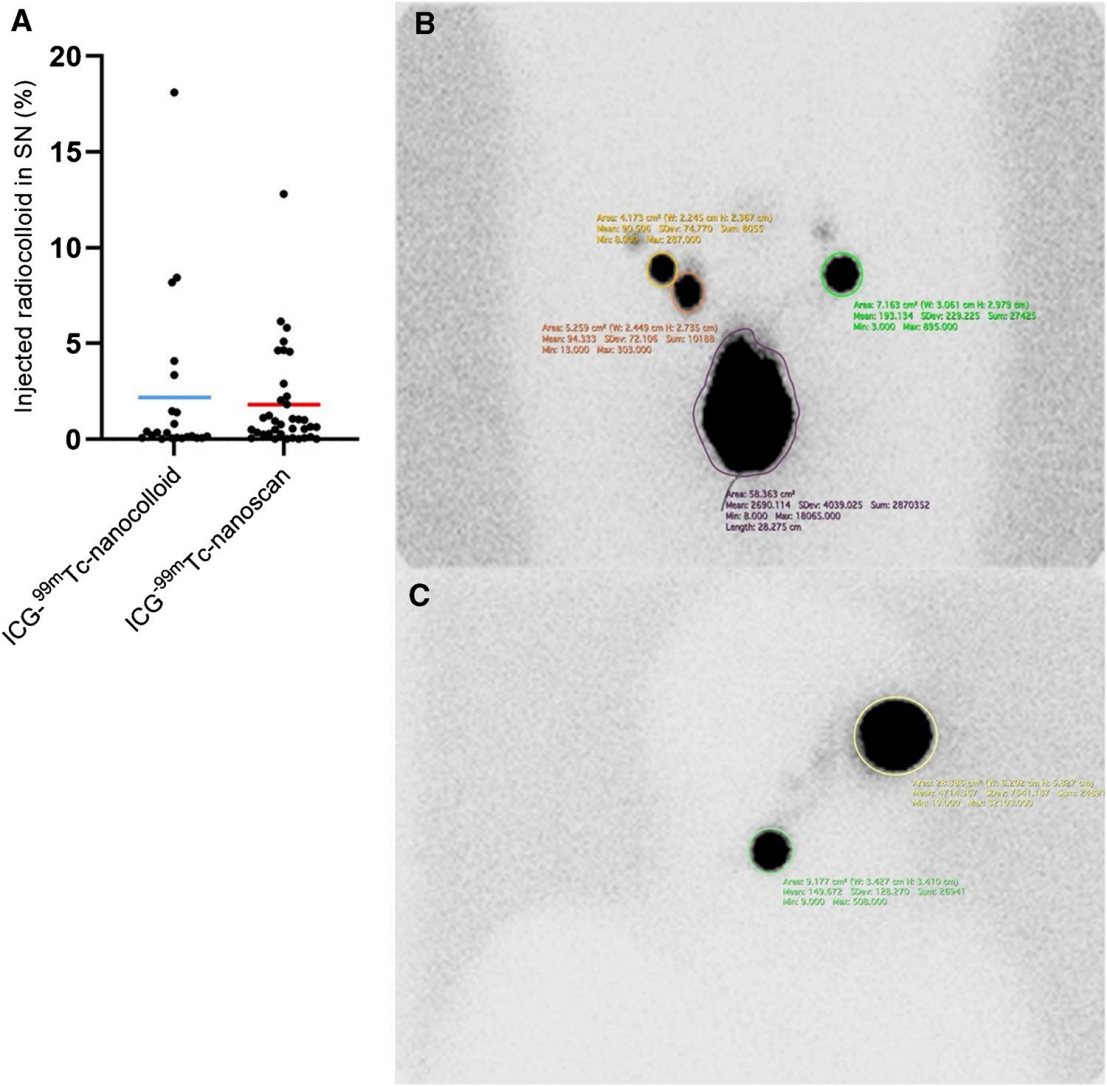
The uptake of injected radiocolloid in SNs on planar lymphoscintigraphy. A There is no difference in uptake when ICG-^99m^Tc-nanocolloid (*blue line*) and ICG-^99m^Tc-nanoscan (*red line*) are compared. B Example of analyses in Horos in a patient with PeCa; ROIs around the injection site (*purple circle*) and SNs (*green, orange and yellow circles*). C And the analysis of a patient with melanoma; ROIs around the injection site (*yellow circle*) and SNs (*green circle*)
